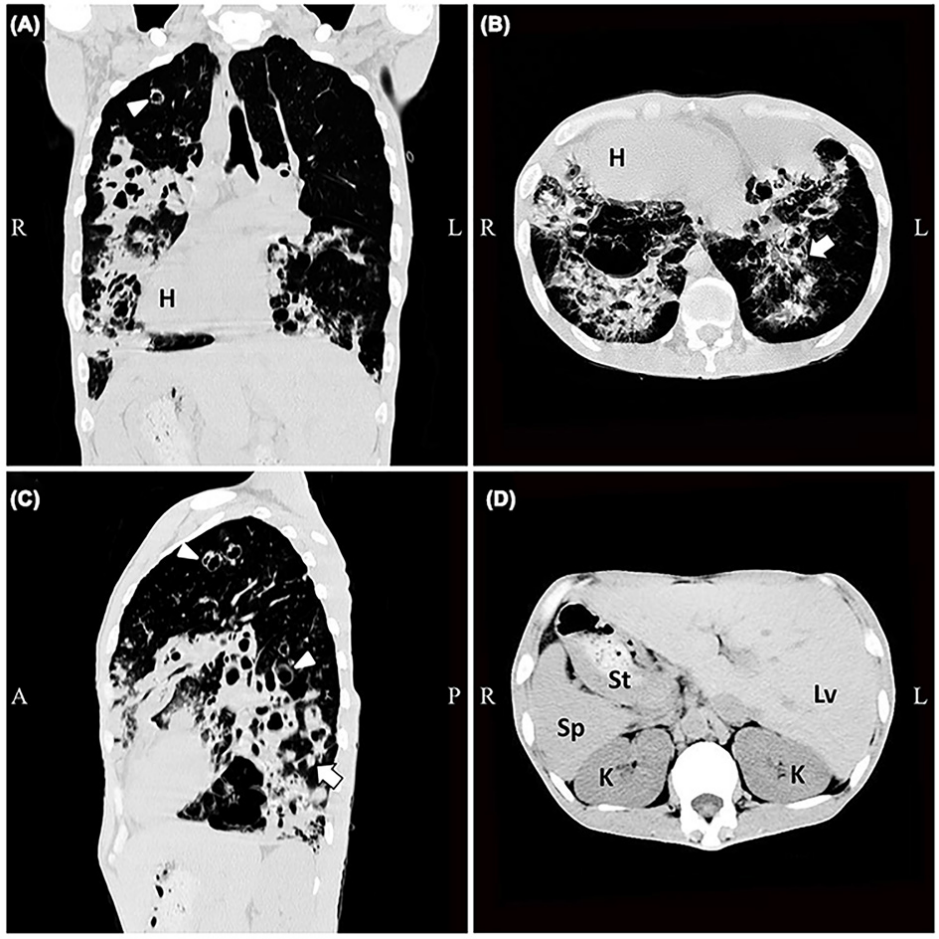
CT scan images of the patient with KS CT scan showed (A) bronchiectasis and right-sided heart, (B) nodules of lungs and right-sided heart, (C) bronchiectasis and nodules of lungs, and (D) spleen and stomach in the right side, and liver in the left side. Dilated bronchi are indicated by arrowheads, and lung nodules are indicated by solid arrows. R: right; L: left; A: anterior; P: posterior; H: heart; St: stomach; Lv: liver; Sp: spleen; K: kidney.
